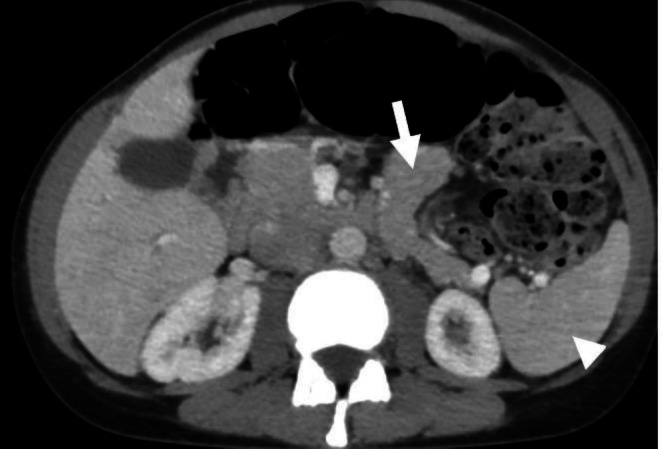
Axial contrast enhanced CT scan. The tail of the pancreas (arrowhead) has returned to a normal anatomic position with normal enhancement and no residual torsion. Similarly, the spleen (arrowhead) has returned to the left upper quadrant without ischemic change, though it remains enlarged.
